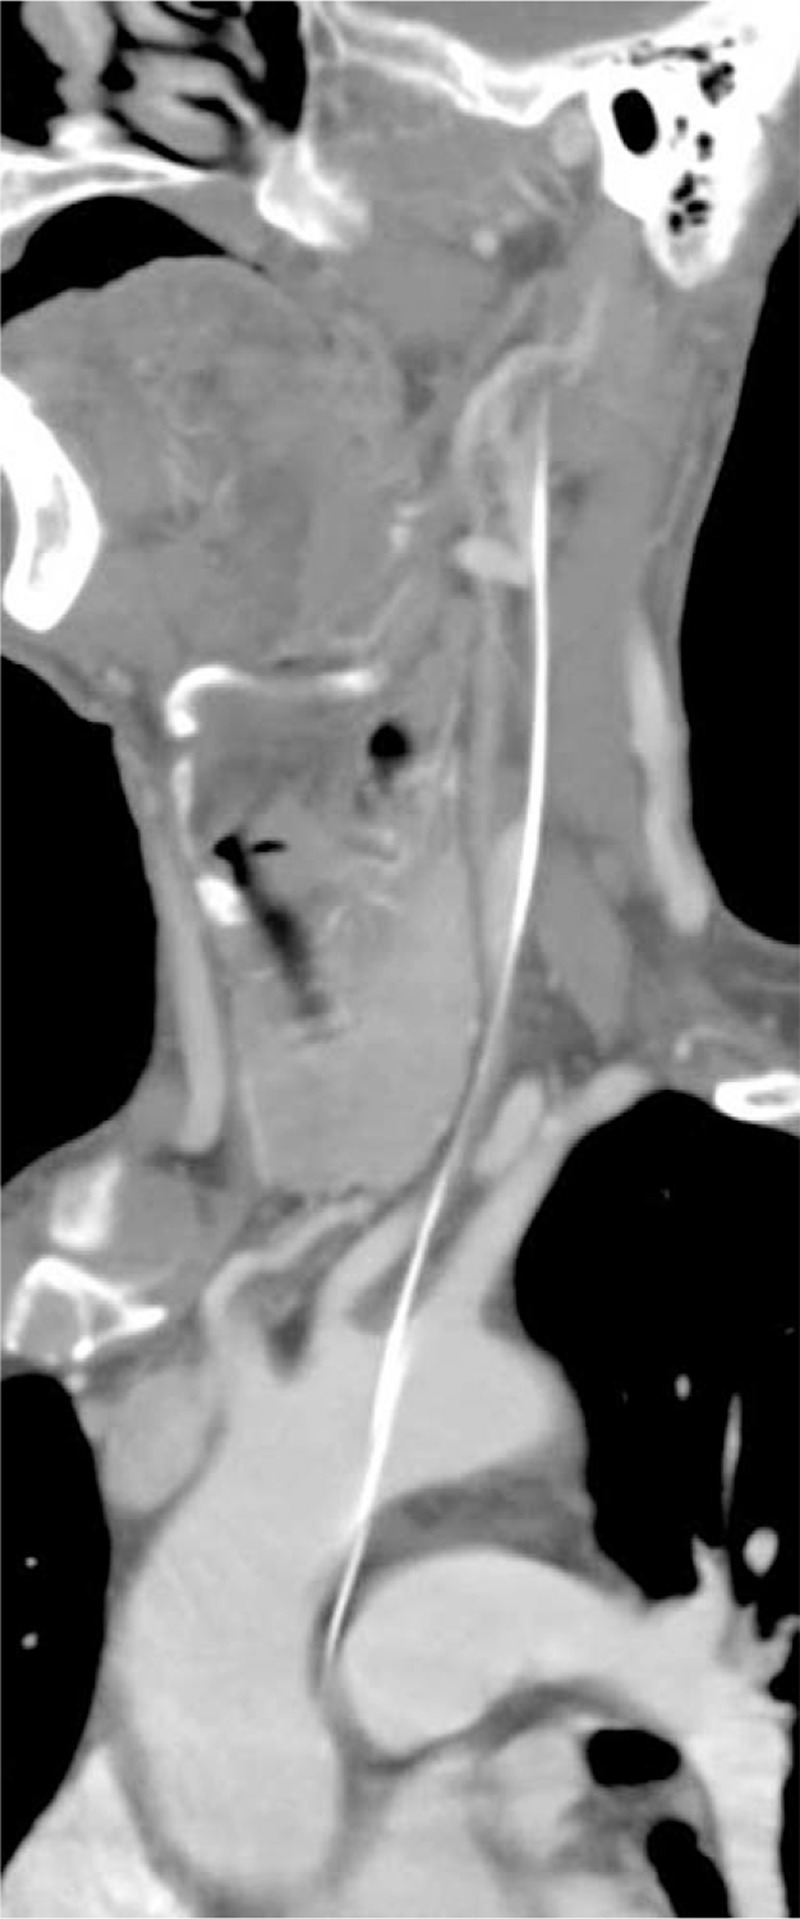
Computed tomography showing guide wire had punctured the vascular wall of the aortic arch to reach the cerebrum.
